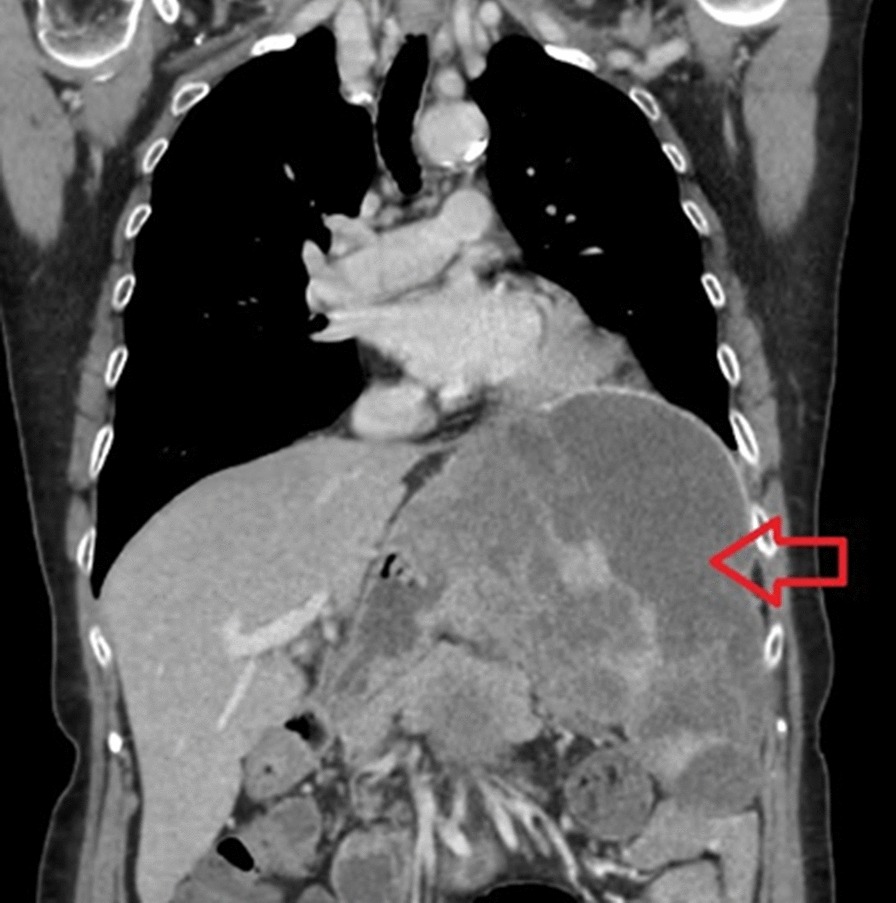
Postoperative CT showing loco-regional failure at the site of the spleen (red arrow)
